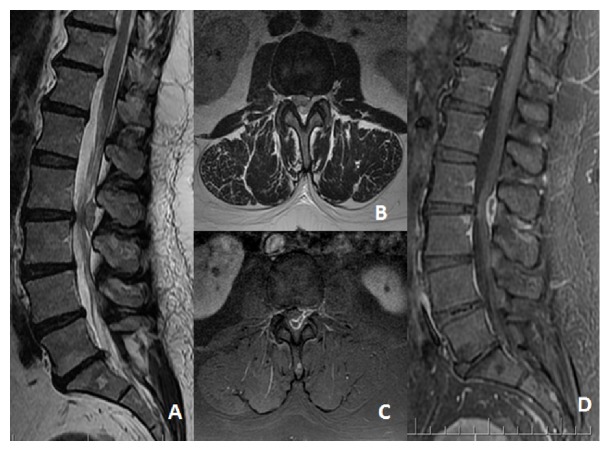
Preoperative MRI scan showing (A) sagittal, (B) axial T2W, (C) axial, and (D) sagittal gadolinium enhanced sequences showing the L2-L3 complete disc extrusion with an anterior-to-posterior circumferential course of the sequestered disc.
